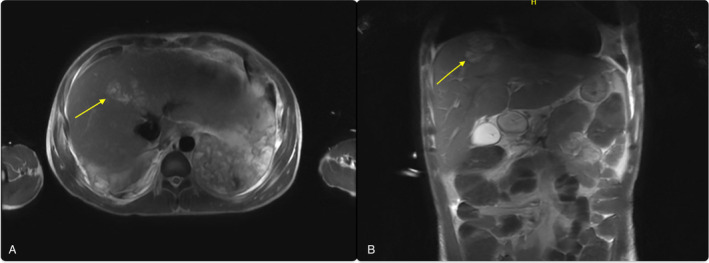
Magnetic resonance imaging (A) Coronal view (B) Sagittal view, showing liver abscess.
